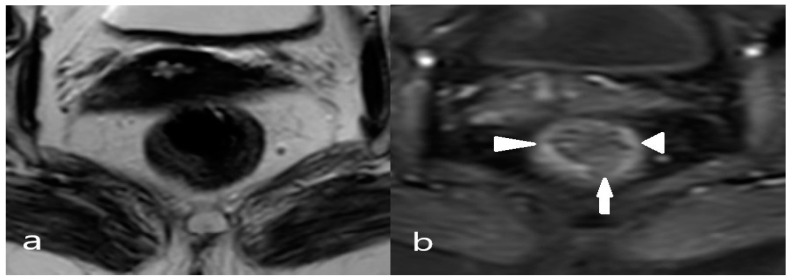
(a) Axial T2WI MRI; (b) Axial contrast-enhanced fat-suppressed T1W MRI: The submucosal stripe is intensely enhancing at the periphery of the tumor (arrowheads). However, it is interrupted at the central base (arrow), suggestive of invasion through the submucosal layer.
